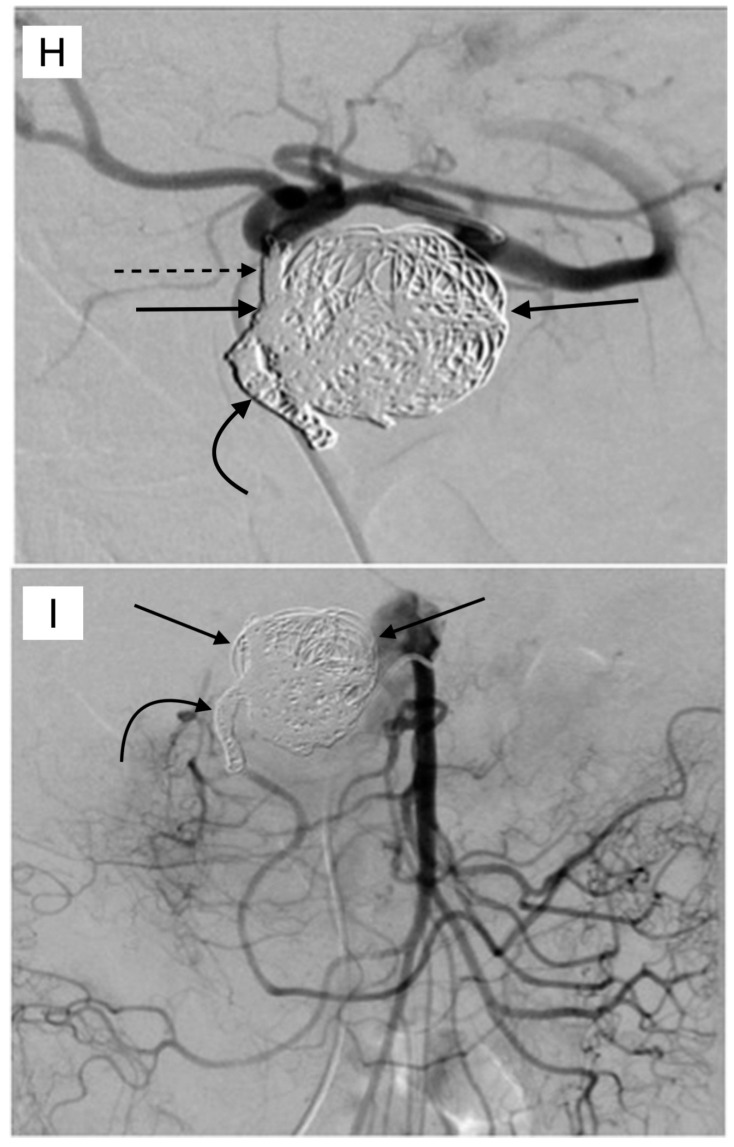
This 75 year old female with chronic pancreatitis secondary to pancreatic divisum who presented with worsening abdominal pain and early satiety. The (A) transverse abdominal ultrasound showing hypoechoic cystic structure (white star), separate from the gallbladder. The (B) color Doppler showed this to be highly vascular (aneurysm or pseudoaneurysm). The (C) T2 weighted abdominal magnetic resonance imaging (MRI) showed the mixed signal intensity within this aneurysm (white arrows). The (D) contrast enhanced imaging in the arterial phase showed the filling of this pseudoaneurysm with some peripheral thrombus. The patient was brought to angiography for an urgent angiogram and embolization. The celiac angiogram in the (E) early arterial and (F) parenchymal phase showed the large pseudoaneurysm (white star) arising from the GDA. The (G) common hepatic artery angiogram showed similar findings. The (H) embolization was then done. The coils were first placed distal to the pseudoaneurysm (curved black arrow), then within the pseudoaneurysm (straight black arrows), and then within the GDA that was proximal to the pseudoaneurysm (dashed black arrow). The (I) superior mesenteric (SMA) injection showed no backdoor filling of the pseudoaneursym. Again, the coils were seen distal to the sac (curved black arrow), as well as within the sac (straight black arrows).
